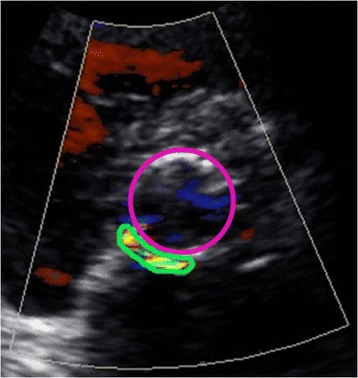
Semi-quantitative echocardiographic (SQE) measurement of paravalvular leak (PVL) using circumferential extent of PVL in the parasternal short-axis by the Valve Academic Research Consortium II method. The green circle represents the circumference of the paravalvular leak. The purple circle represents the circumference of the transcatheter valve stent frame. The circumferential extent is the sum of the paravalvular leak jet circumference(s) divided by the valve circumference (mild <10%, moderate 10-30%, severe >30%).
